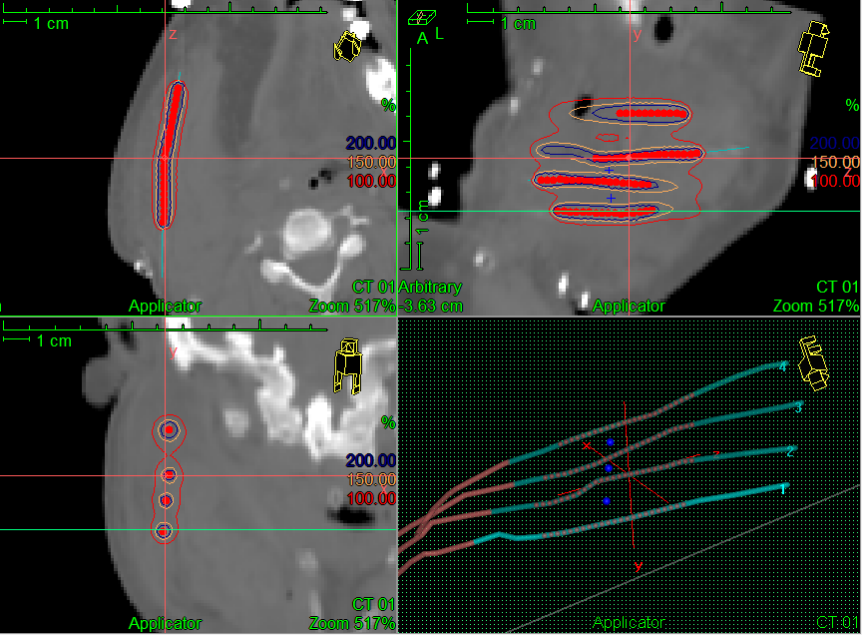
CT cross section image from Treatment Planning System showing reconstructed catheters
